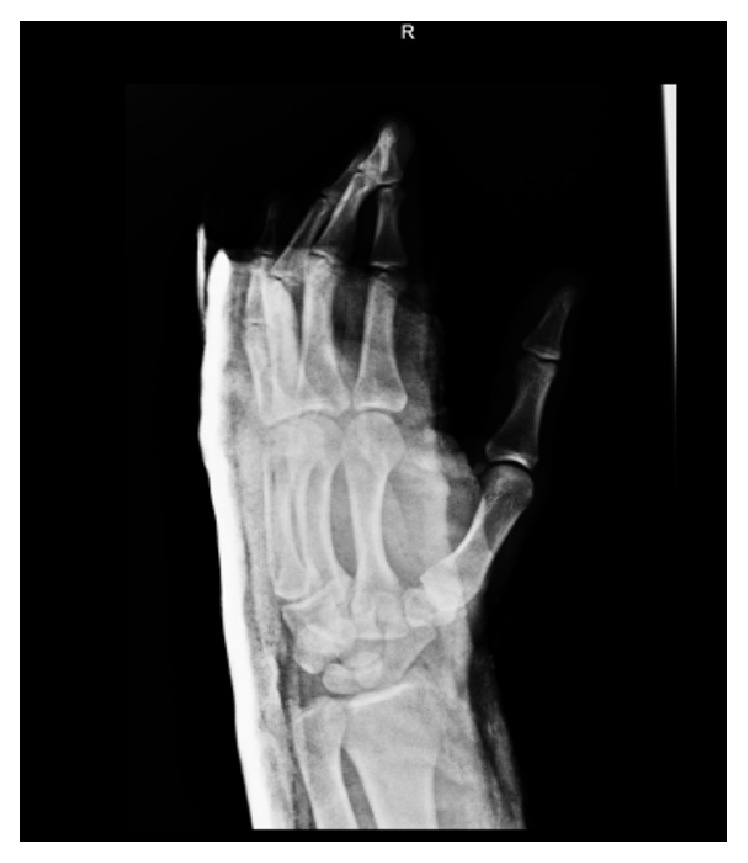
After reduction X-ray: optimal reduction, no more signs of dislocation.
